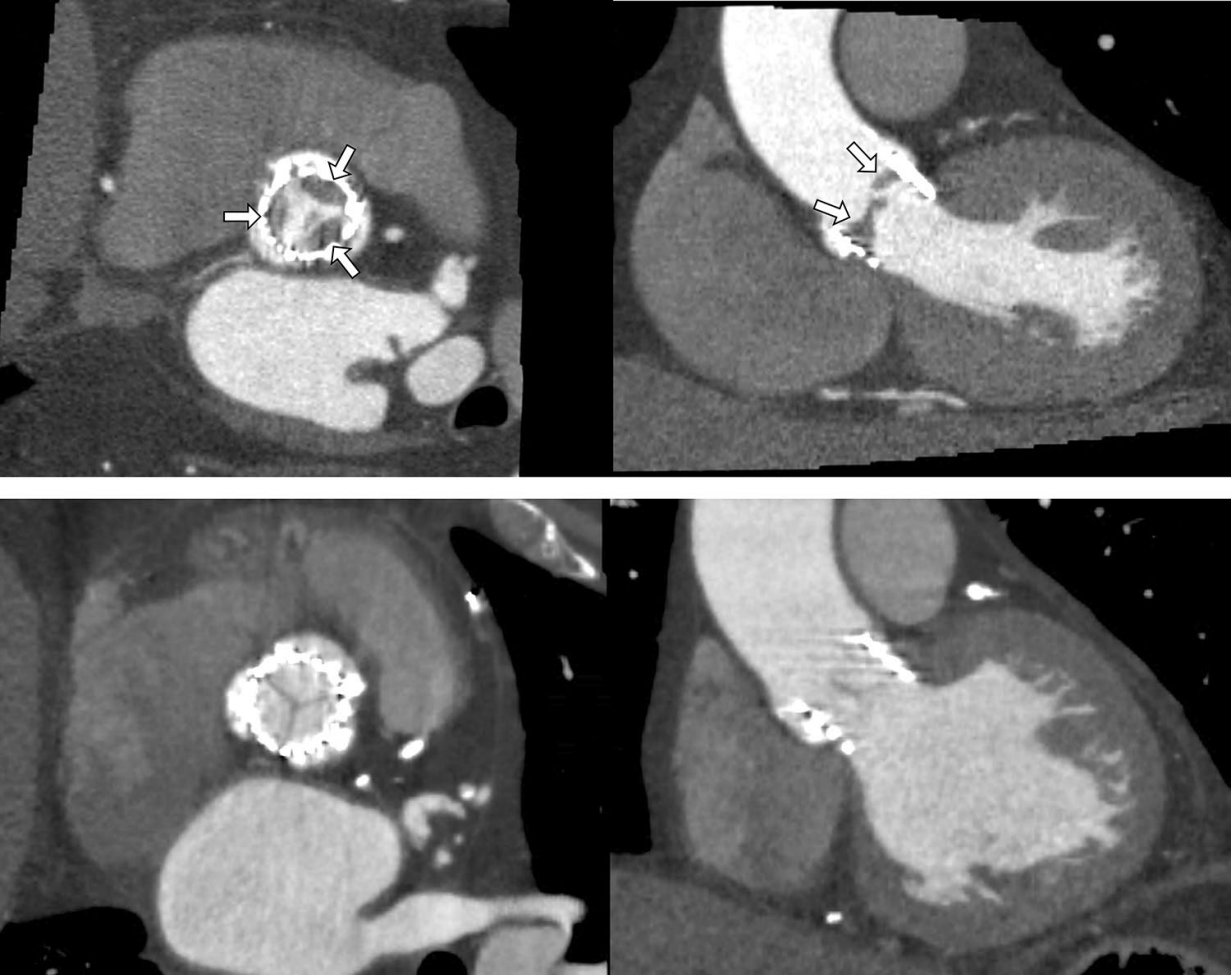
Multiplanar reconstructions of transcatheter aortic valves on multidetector row computed tomography with HALT (white arrows, upper panel) and without HALT (lower panel)
